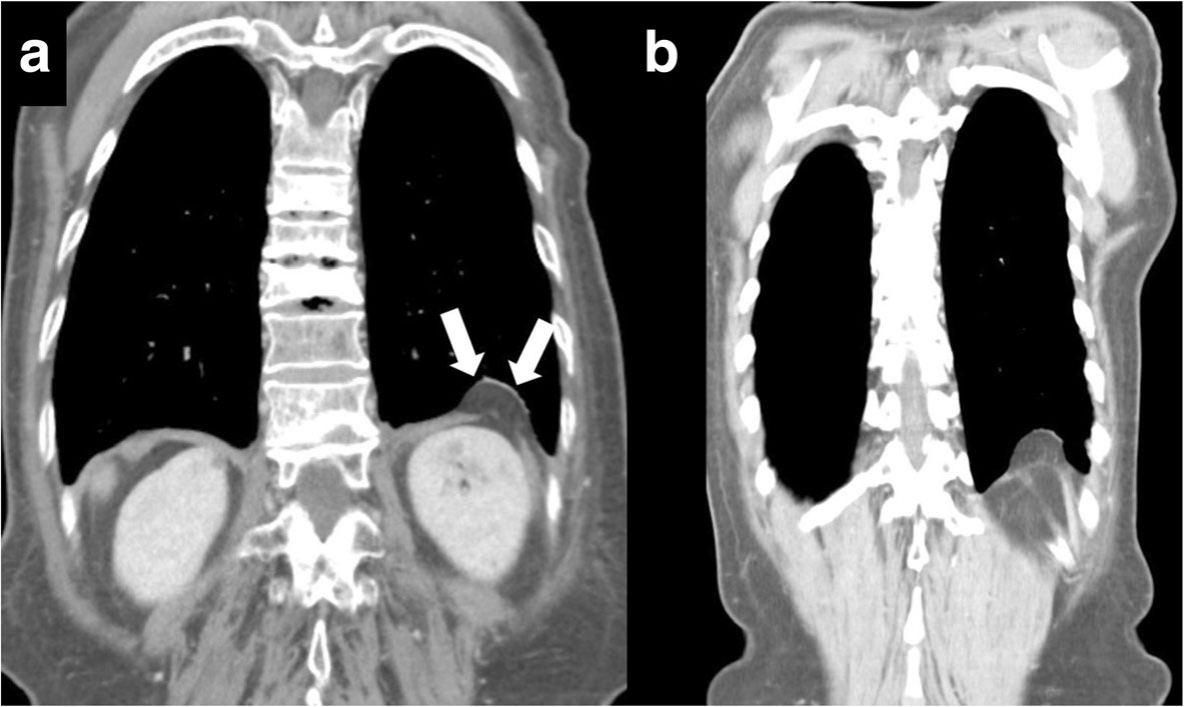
a Computed tomography (CT) revealed a defect in the left diaphragm and herniation of retroperitoneal fat tissue (3 × 1.3 cm) into the left thorax (*white arrows*). The size of hernia has not changed compared with the size observed on past CT scans. b Past computed tomography finding (1 year and 3 months before the scan obtained in Fig. 2a)
